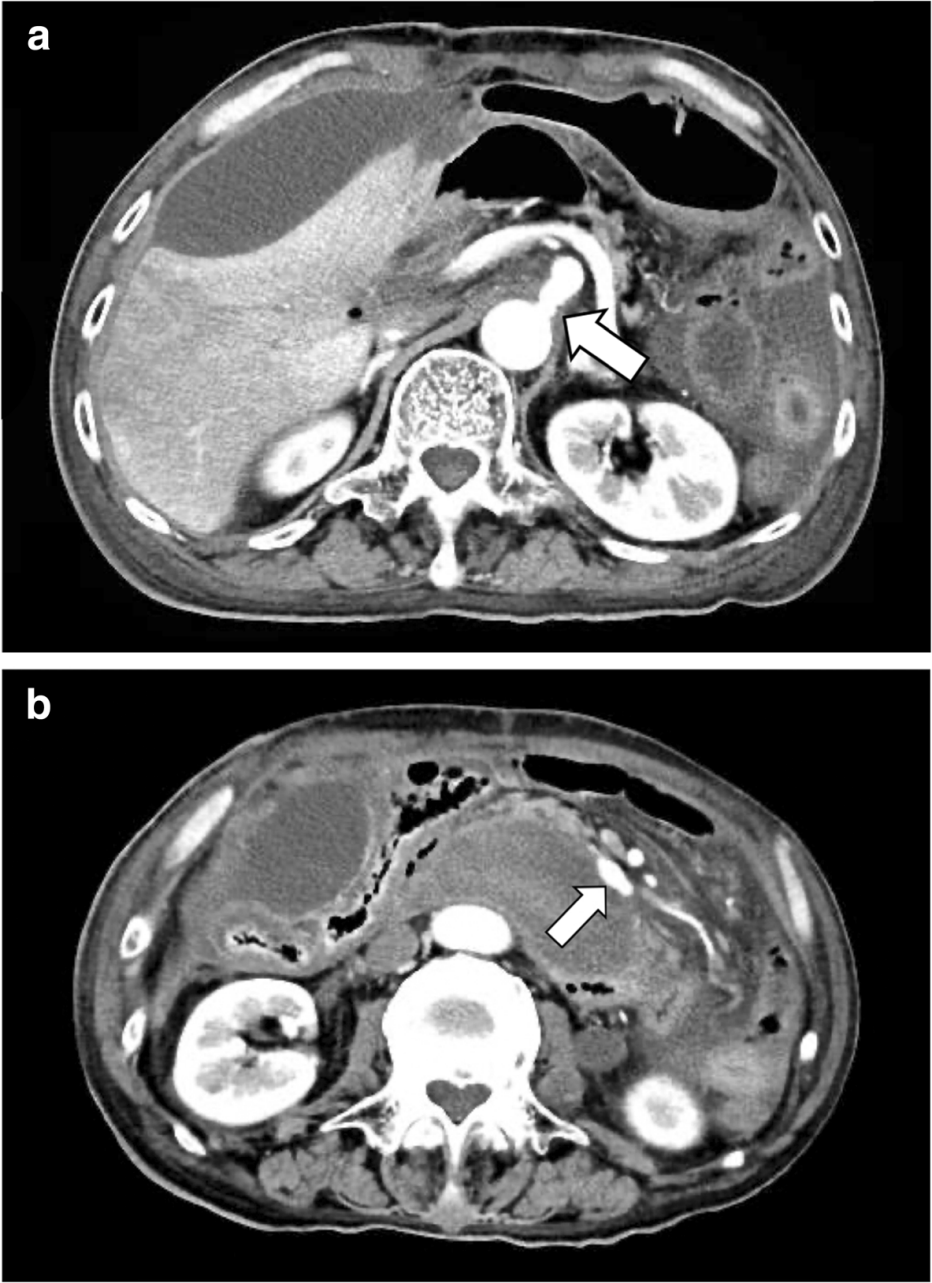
a The abdominal computed tomography on postoperative day 4 determined that the arterial diameter of the celiac axis has expanded slightly (shown by arrow). b The abdominal computed tomography on postoperative day 4 determined that there was no significant change in the size (13 × 7 mm) of the untreated aneurysm of the anterior inferior pancreaticoduodenal artery (shown by arrow)
